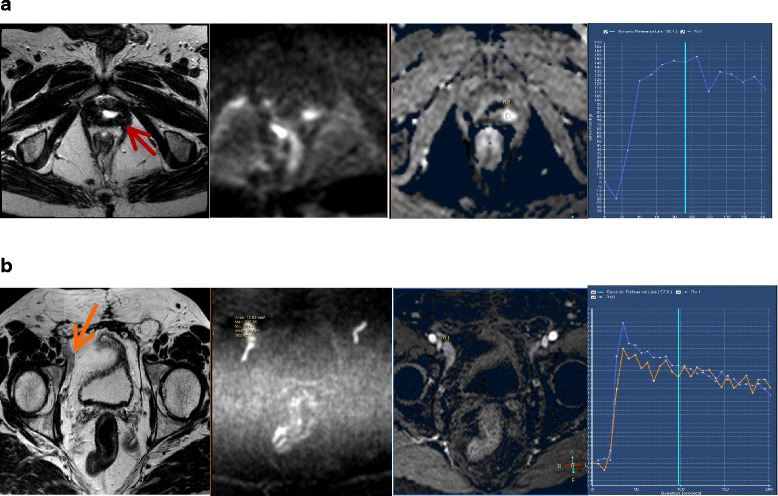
mpMRI malignancy criteria for local recurrence (a) and lymph node (b) recurrence. From left to right: T2-weighted images, DWI and dynamic contrast enhance image amb curve
